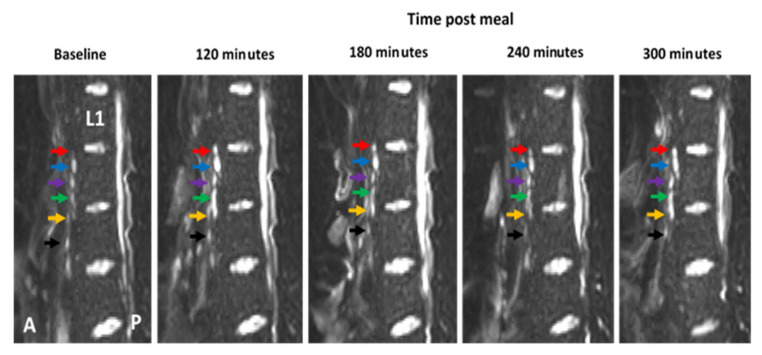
Example of diffusion weighted image (DWI) showing how the vertebra of the spine can be used as an anatomical marker to allow for the same lymph nodes to be consistently imaged at multiple time-points pre- and post-meal. The first lumbar vertebra is indicated (L1) and lymph nodes are indicated by coloured arrows. Colours represent the same individual lymph at each time point. The anterior side (A) of the body is to the left of each image and the posterior side (P) is on the right.
